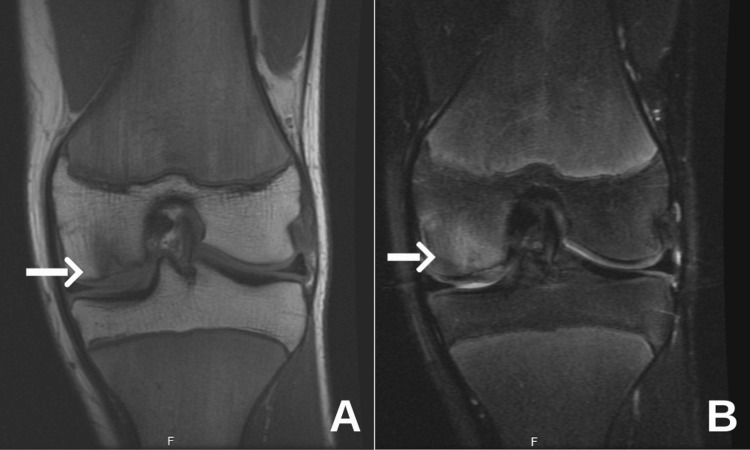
Coronal MRI images of the left knee. (A) The T1-weighted image shows a persistent osteochondral defect (arrow). (B) The corresponding T2-weighted image shows a decrease in bone marrow edema (arrow). MRI, magnetic resonance imaging
